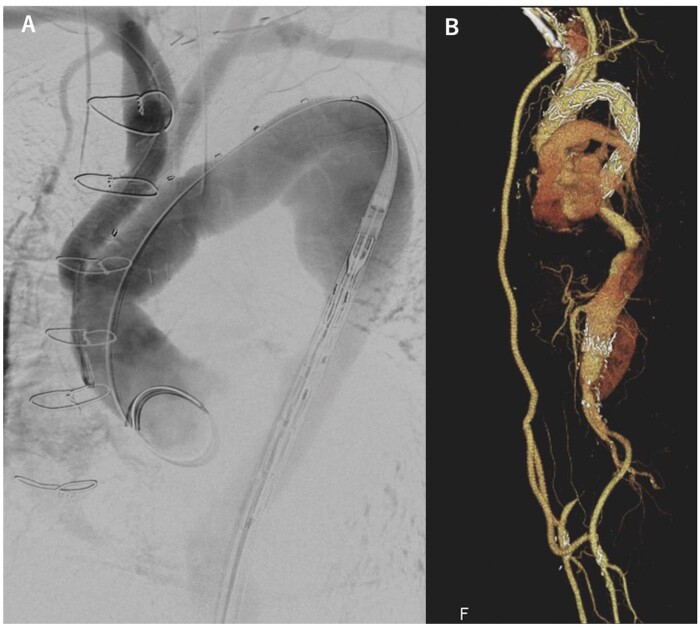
Total arch replacement with elephant trunk followed by thoracic endovascular aortic repair. (A) Intraoperative image of secondary thoracic endovascular aortic repair after total arch replacement with elephant trunk. (B) Postoperative 3D computed tomography image after thoracic endovascular aortic repair.
